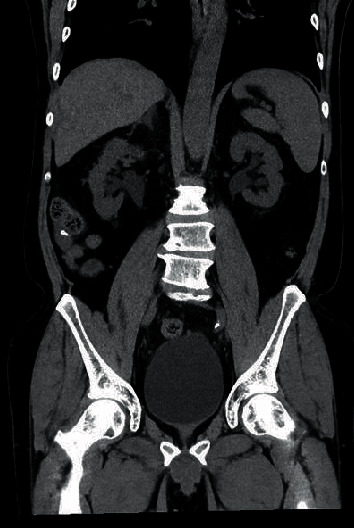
Noncontrast CT abdomen in coronal plane showing bilateral hydronephrosis.
